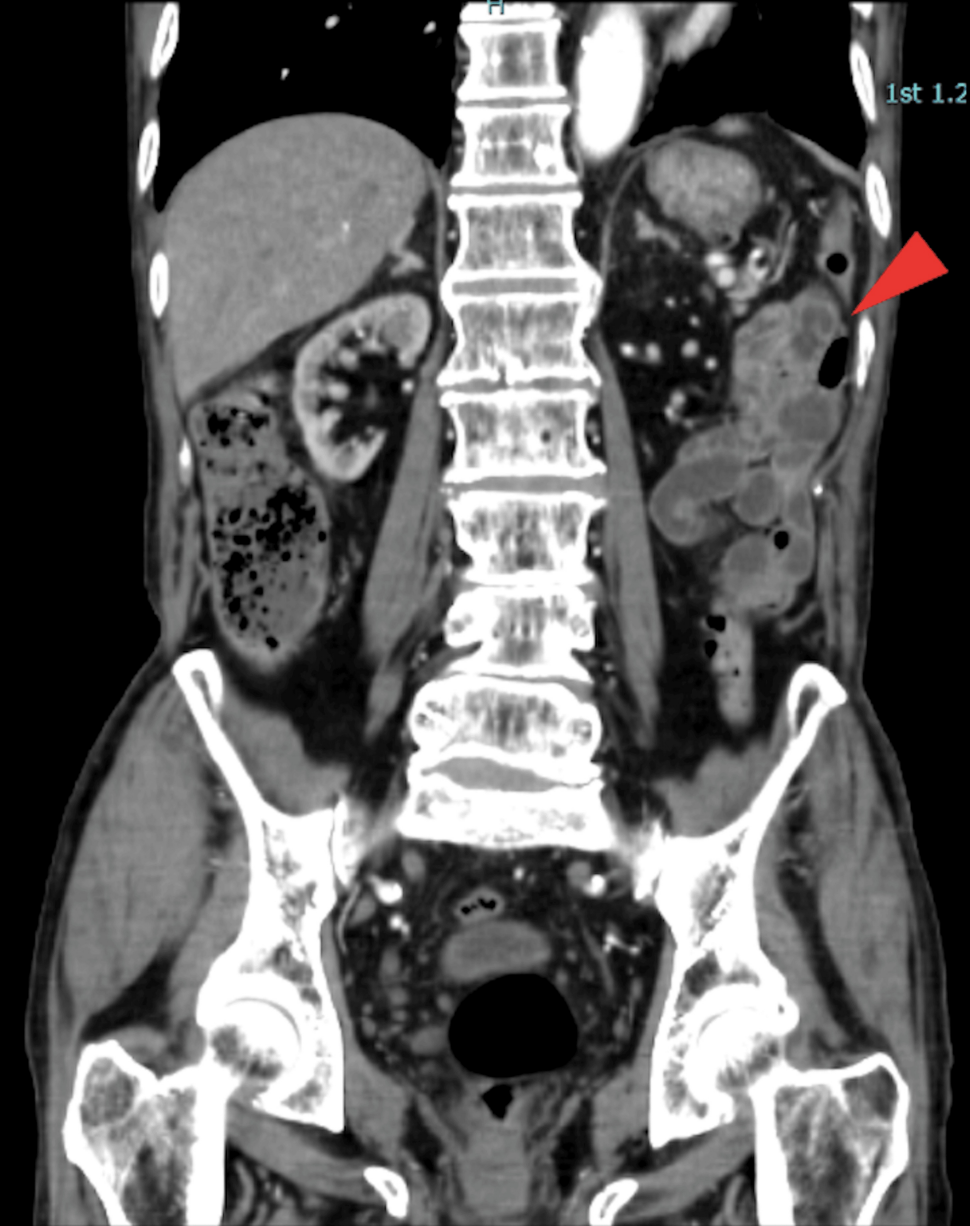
Contrast-enhanced abdominal CT (coronal view) Segmental thickening of the left colon wall with increased perienteric fat density (arrowhead) is observed.
